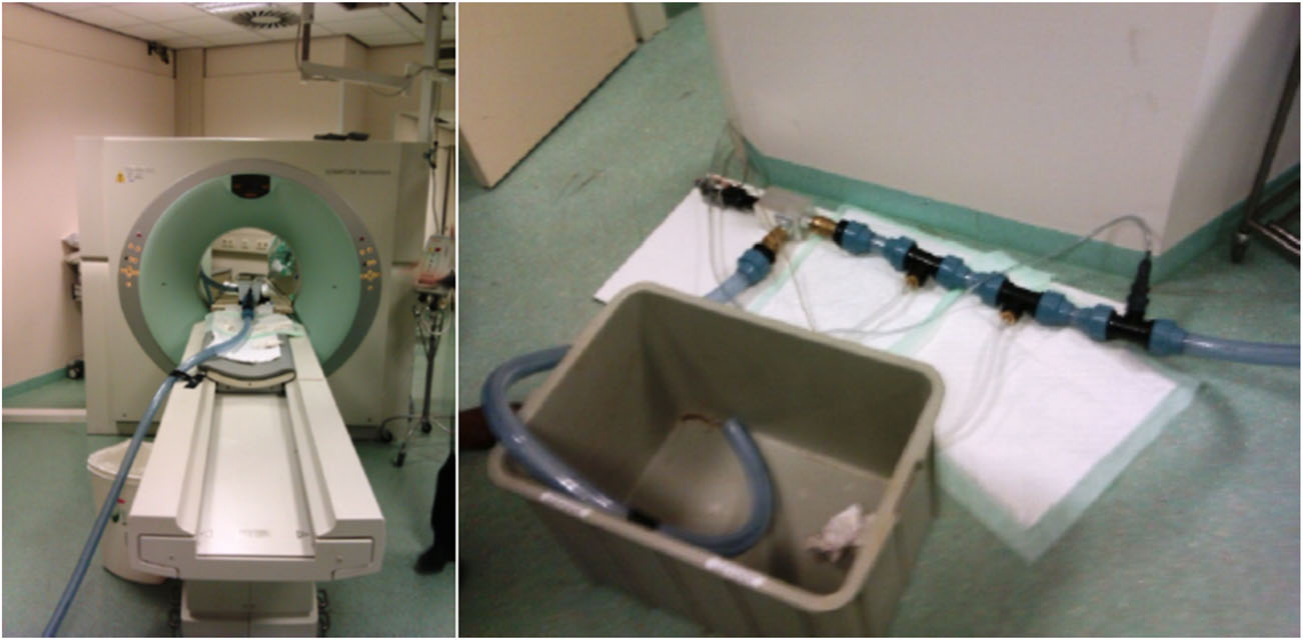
The testing with Computed Tomography
